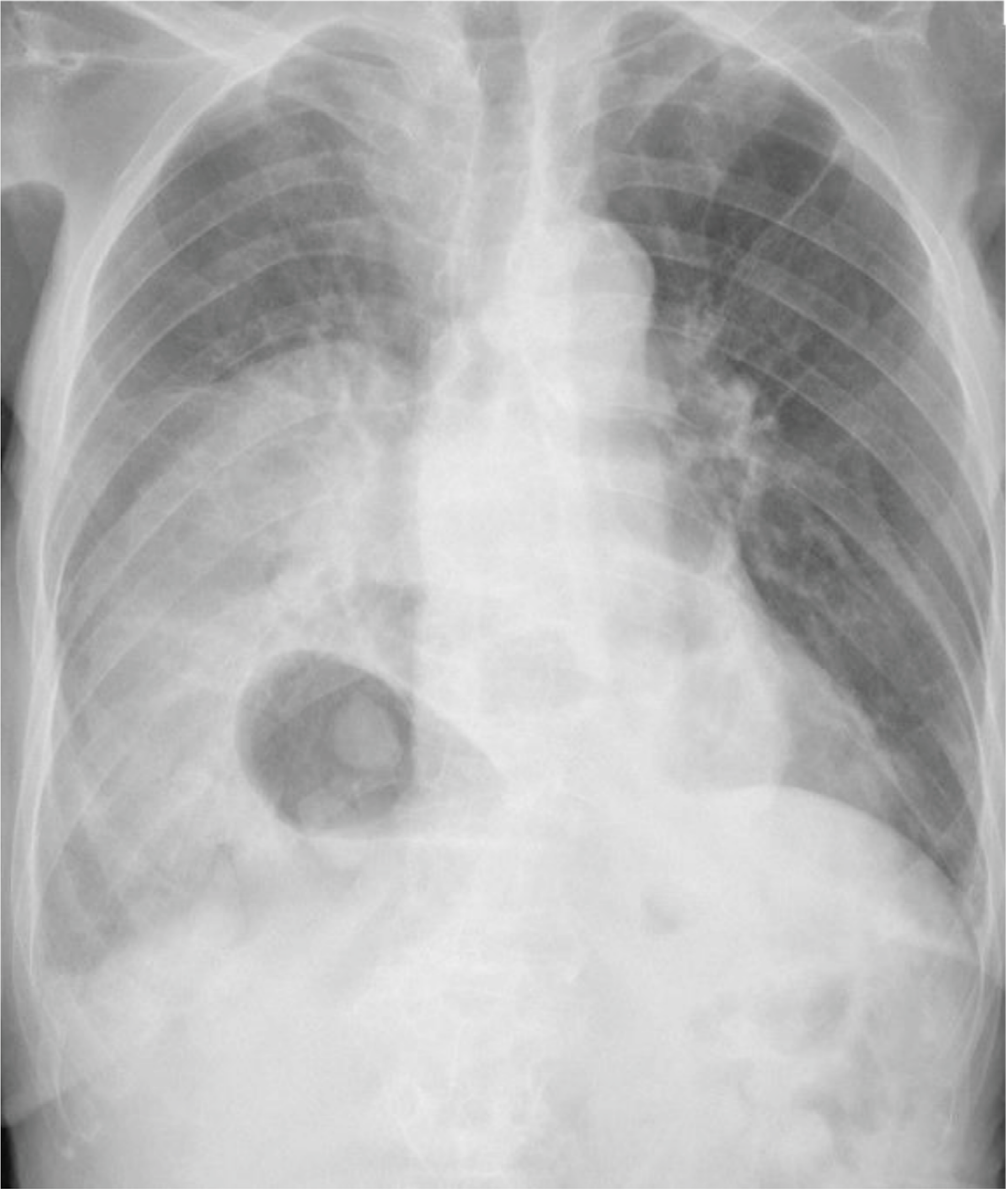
Frontal chest X-ray demonstrates multiple cystic lucencies within the right hemithorax, with an indistinct right hemidiaphragm and relative paucity of bowel gas in the upper abdomen. The left lung field appears clear.
